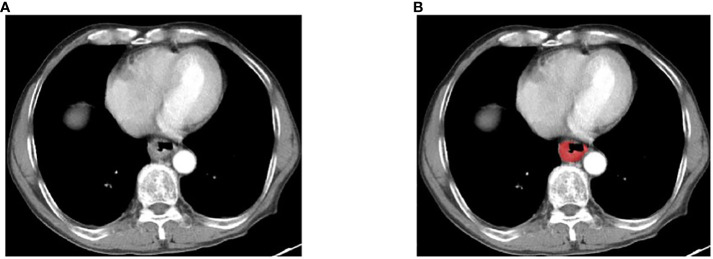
An example of manual segmentation in ESCC. (A) Localized thick wall of esophageal cancer with enhancement is observed on the arterial phase computed tomography (CT) image; (B) Manual segmentation on the same axial slice is depicted with red label.
